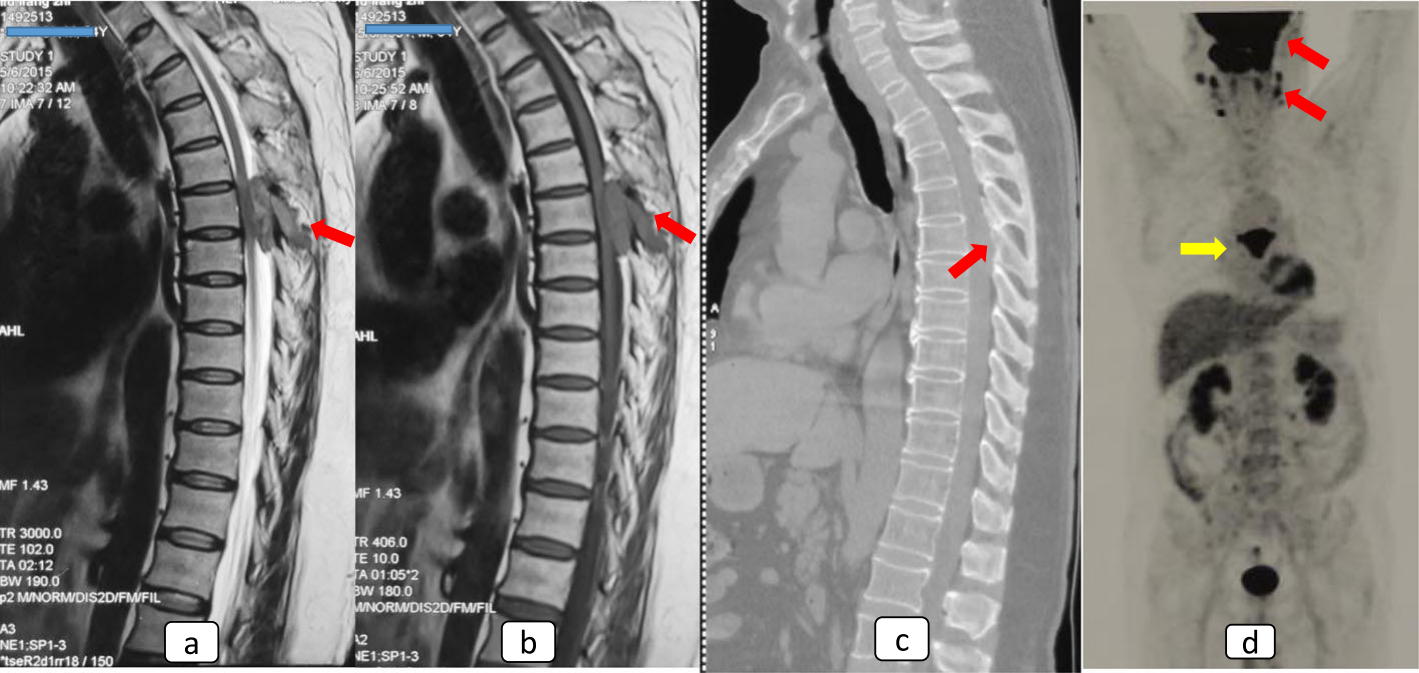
The imaging presentation of a case with spinal RDD. MRI scans manifested a T1 isointense (a) and T2 slightly hyperintense (b) extradural lesion on Th6. The spinous process was also involved (arrows on a and b). CT scan revealed an osteolytic lesion, but the cortex seemed intact (arrow on c). PET/CT scan was arranged. Paranasal lesions and cervical lymph node lesions were also found and the lesions manifested a high uptake of 18-FDG (red arrows on d)
